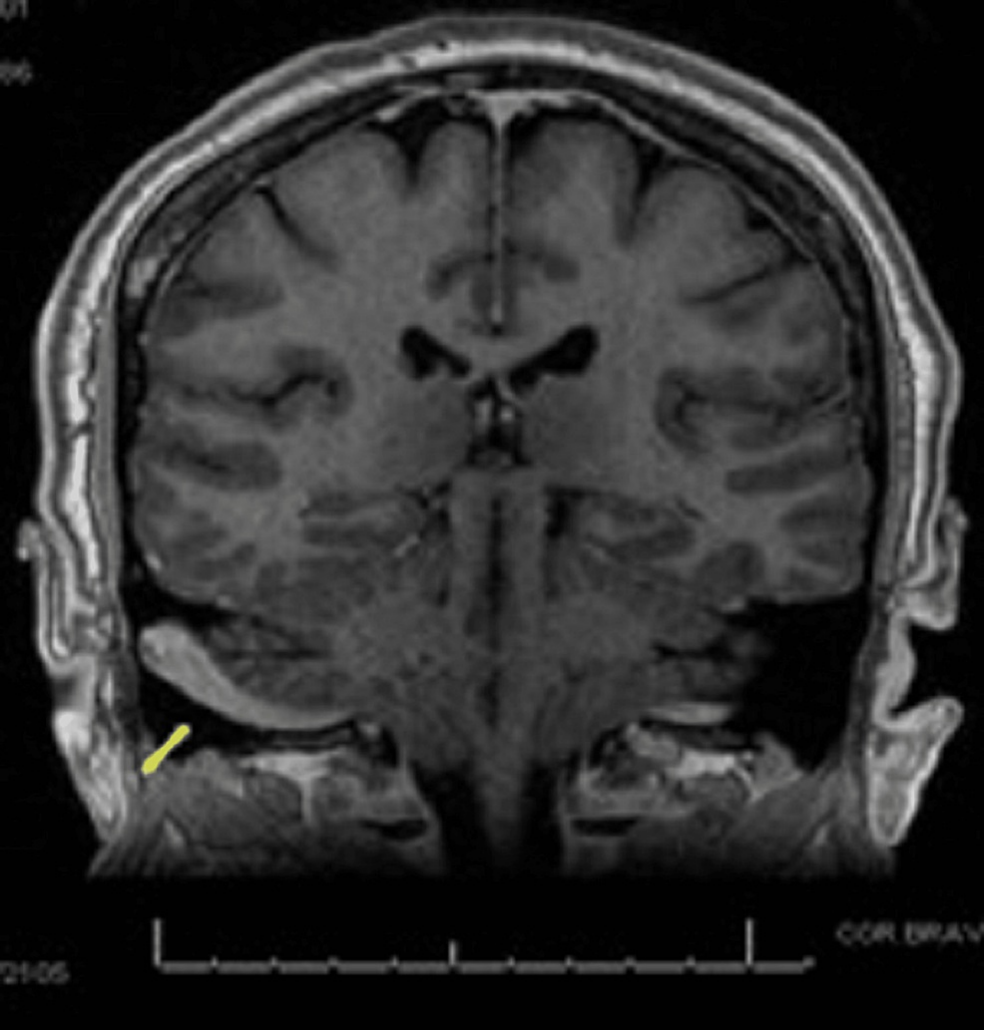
Coronal view of T1-weighted brain MRI revealing dominant right-sided sigmoid sinus (arrow) extending laterally toward the cortex of the mastoid bone.
